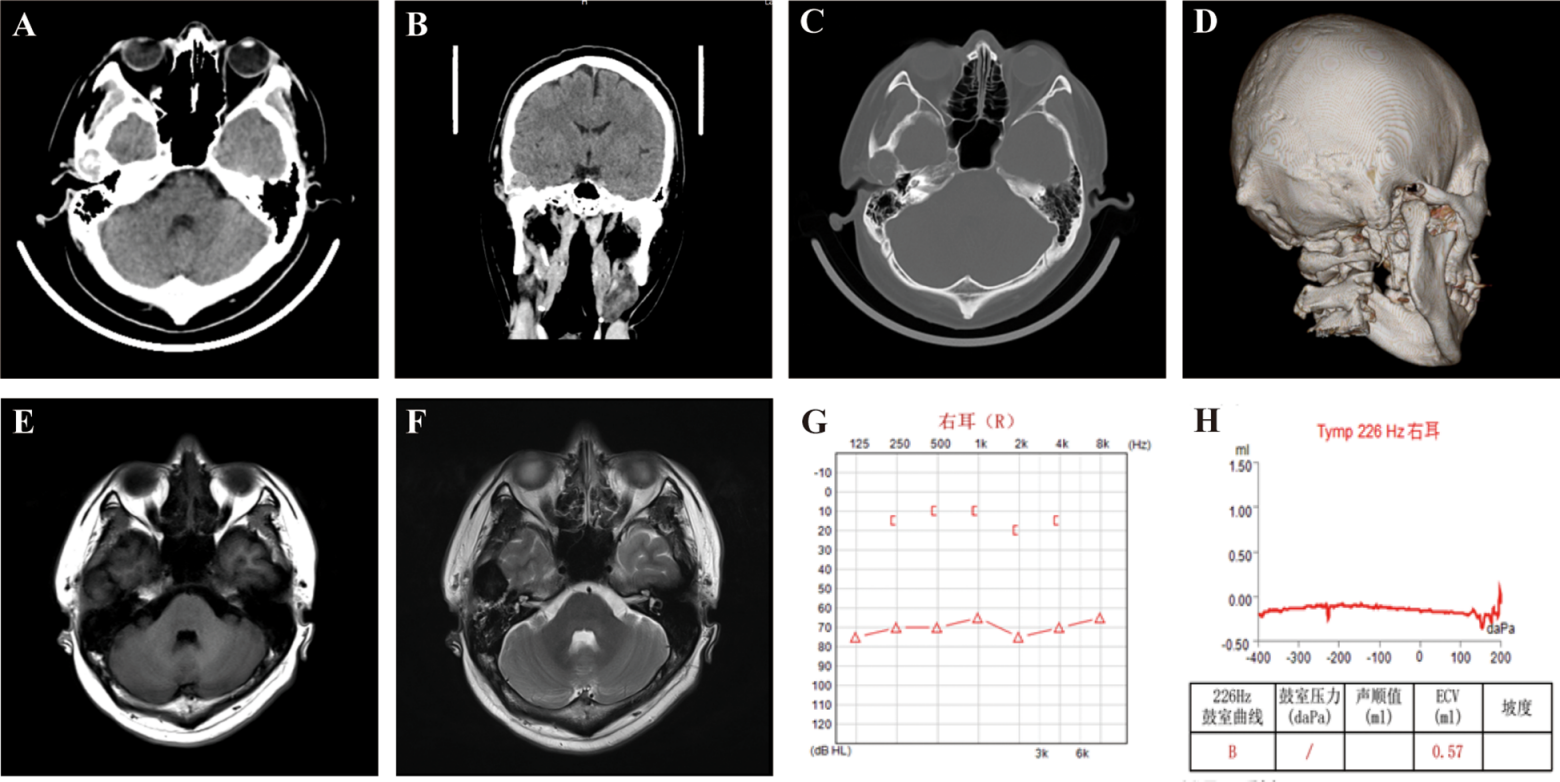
Preoperative imaging and audiological assessments of the patient demonstrating a right temporal bone mass and associated hearing impairment. (A–D) Axial and coronal CT (A, B), bone window (C), and 3D reconstruction (D) show osteolytic bone destruction in the right temporal bone with a soft tissue density mass, measuring approximately 17 × 14 mm. (E, F) MRI reveals a well-defined, lobulated lesion in the right temporal region, approximately 2.3 × 2.8 × 3.1 cm in size. The lesion shows iso- to slightly hypointense heterogeneous signals on T1-weighted imaging (E), hypointense signals on T2-weighted imaging (F). Adjacent brain parenchyma is compressed and displaced, and adjacent bone shows thinning and resorption. (G) Pure-tone audiometry indicating severe sensorineural hearing loss in the right ear across all tested frequencies. (H) Tympanometry of the right ear showing a Type B curve (flat), indicating middle ear effusion or dysfunction.
